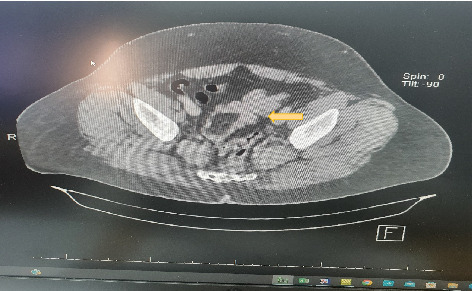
CT scan abdomen showed proximal colon dilatation with distal collapse.
